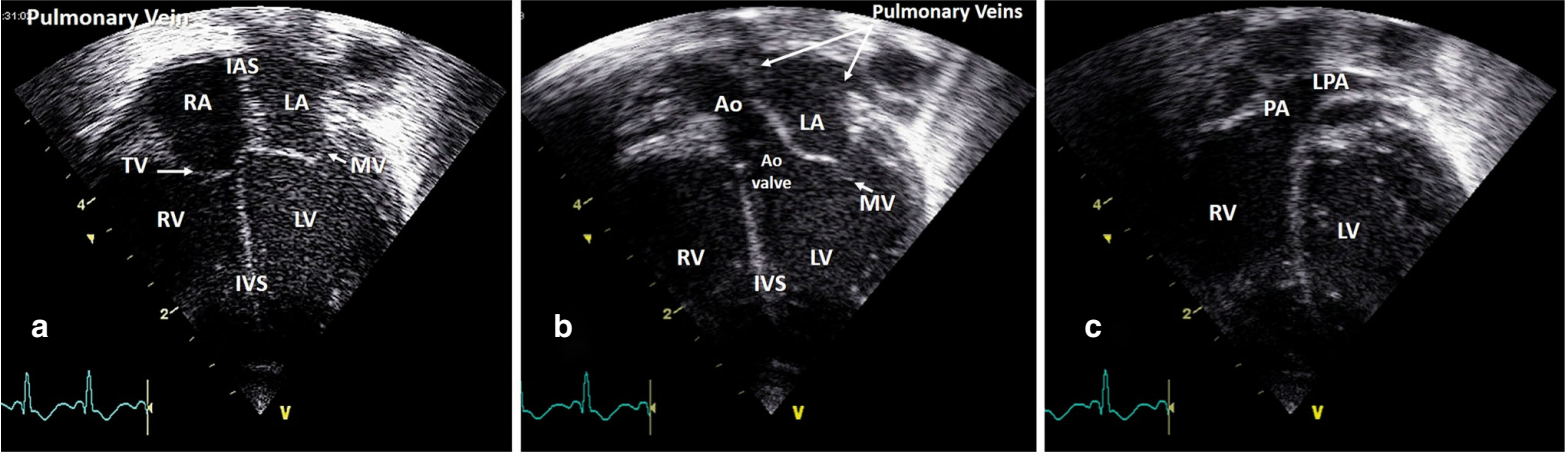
Apical four-chamber view (a), LV outflow tract (b), and RV outflow tract (c). IAS interatrial septum, IVS inter-ventricular septum, Ao aorta, LA left atrium, LV left ventricle, MV mitral valve, RA right atrium, RV right ventricle, TV tricuspid valve, LPA left pulmonary artery
